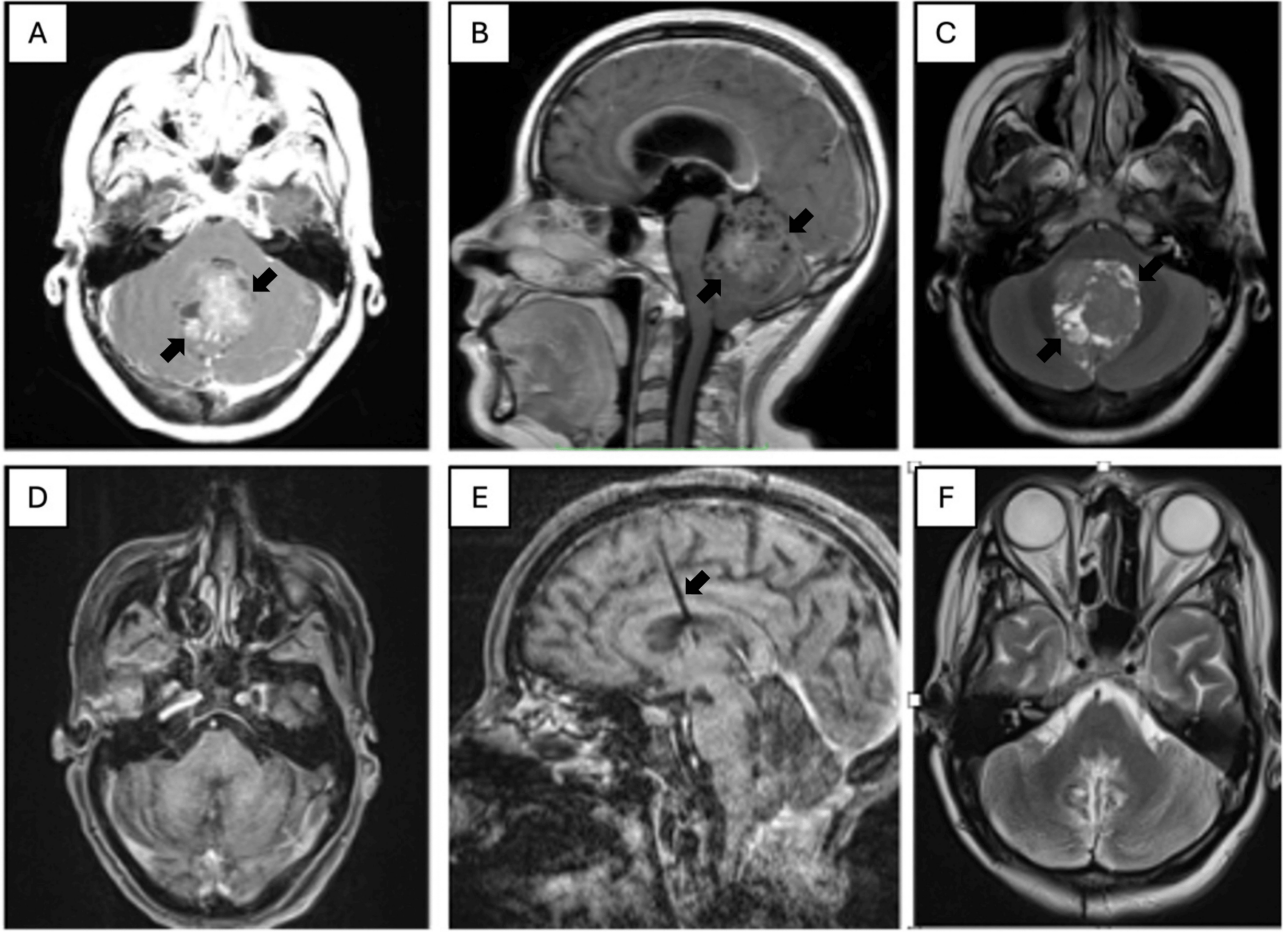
Magnetic resonance imagining reports A. Axial in contrast-enhanced T1 sequence showing a lesion dependent on the fourth ventricle (arrows) with heterogeneous enhancement to the contrast medium. B. Sagittal in contrast-enhanced T1 sequence revealing a lesion dependent on the roof of the fourth ventricle (arrows), with heterogeneous enhancement to the contrast medium, and collapse of the ventricular system with evidence of lateral ventricle dilation. C. Axial in T2 sequence showing a lesion with heterogeneous solid and cystic areas, with probable calcifications inside (arrows). D. Axial in contrast-enhanced T1 sequence demonstrating total resection of the lesion, with adequate expansion of the cerebellar hemispheres. E. Sagittal in contrast-enhanced T1 sequence showing the fourth ventricle without evidence of neoplastic lesion, with postsurgical changes secondary to resection, and a precoronal ventriculoperitoneal shunt (arrow), with no signs of hydrocephalus. F. Axial in T2 sequence showing no evidence of residual lesion, with no cerebellar or brainstem lesion detected.
